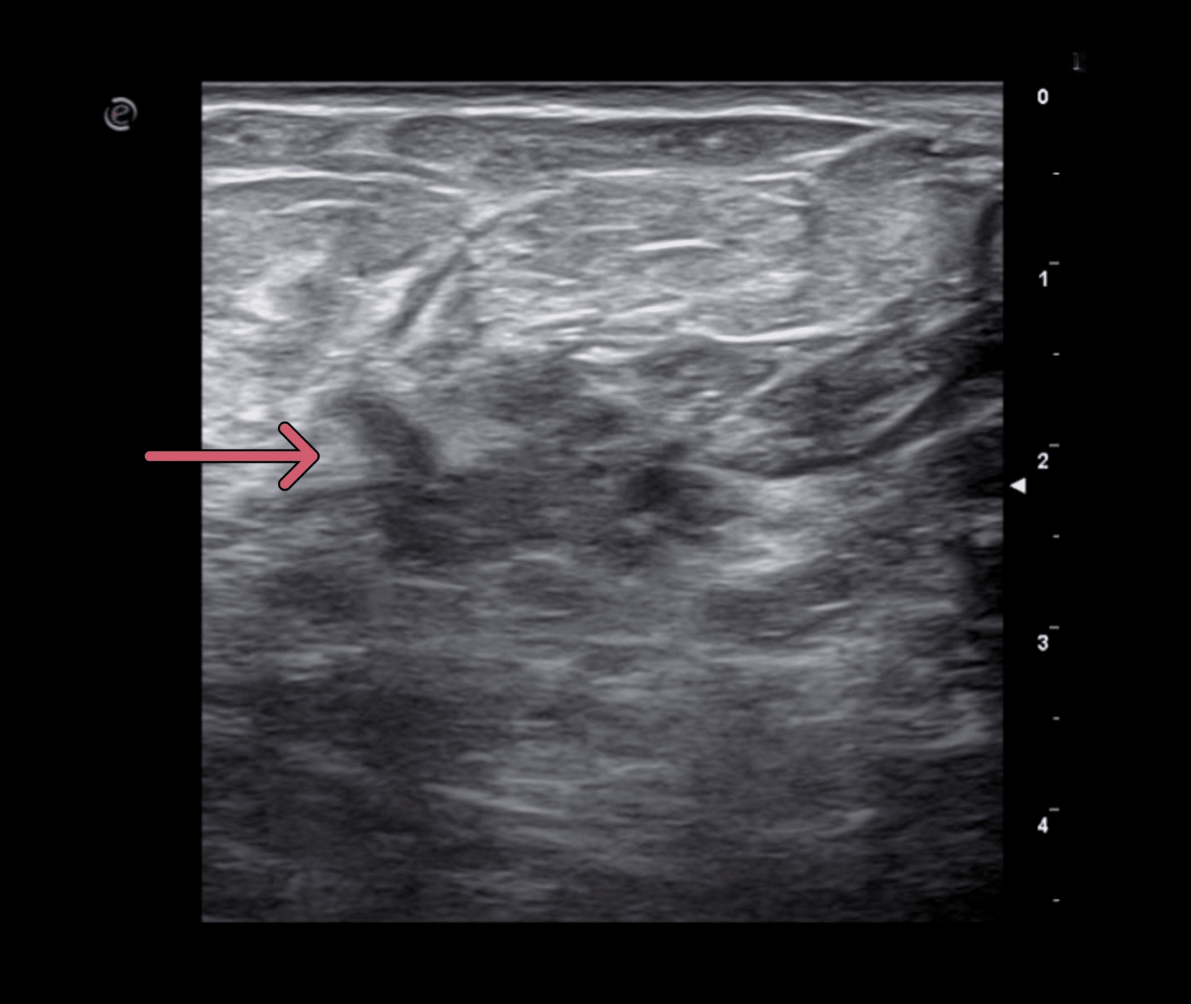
Breast ultrasound. Breast ultrasound reveals an irregular area of altered parenchymal echogenicity, with hypoechoic tissue showing infiltrative growth extending from the peri-areolar region to the outer quadrants (the arrow indicates the area of interest).
